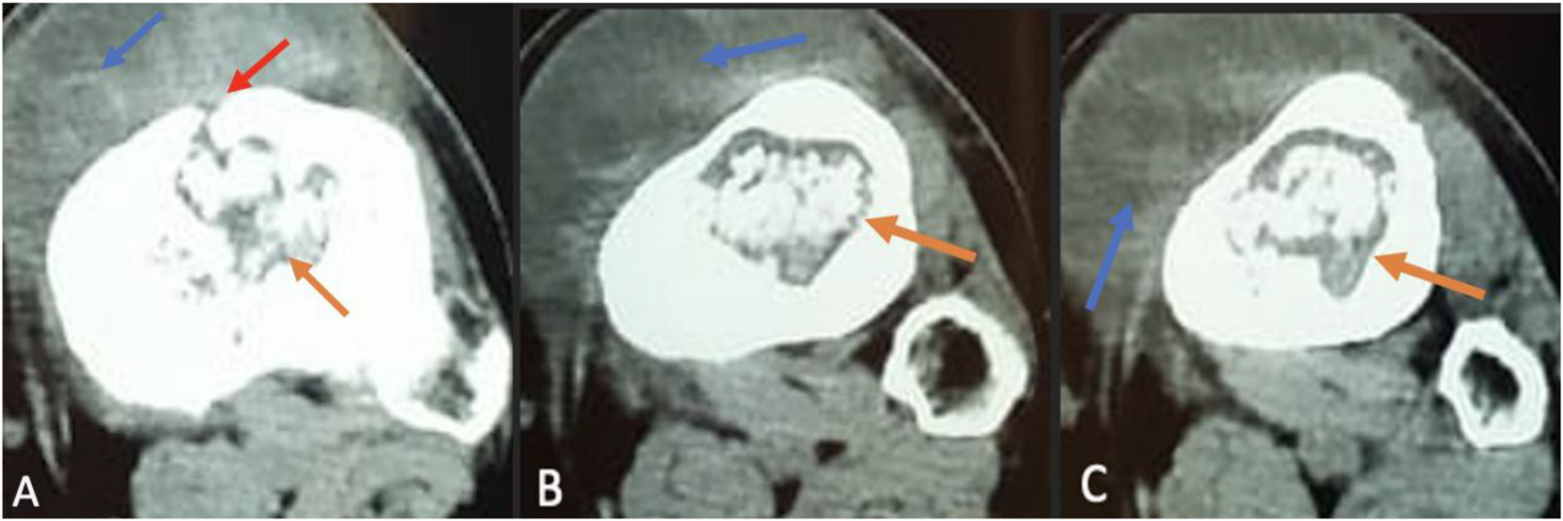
Axial CT scans without contrast injection show a well-defined lytic lesion (orange arrow) in the proximal left tibia, with anterior cortical breach (red arrow) and adjacent cortical destruction, communicating with a hypodense pre-tibial soft-tissue collection (blue arrow). A surrounding rim of intramedullary sclerosis is also visible, consistent with tuberculous osteomyelitis with associated soft-tissue abscess.
